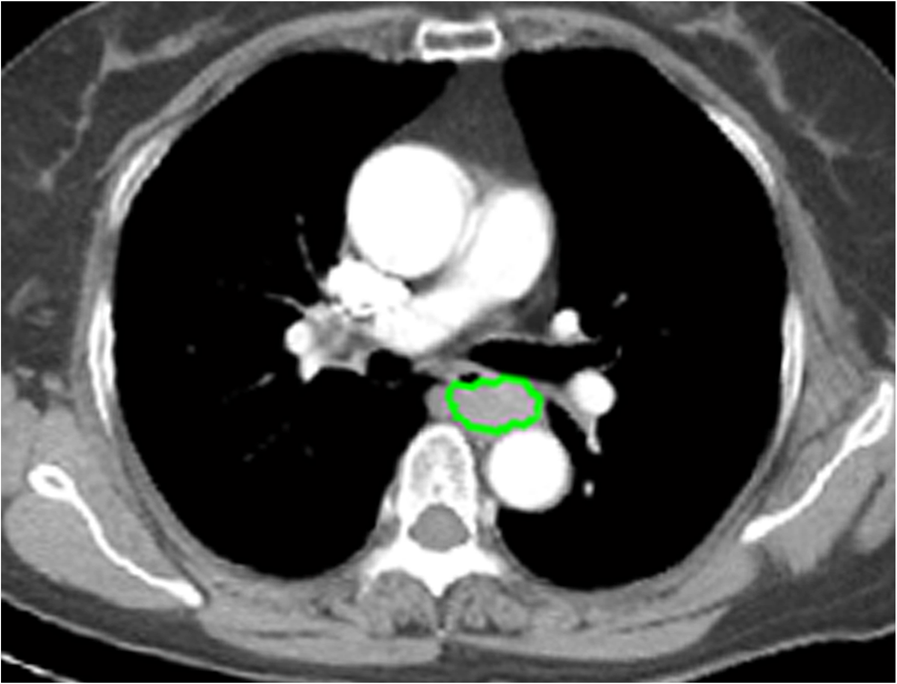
The outlines of oesophageal cancer are manually drawn slice by slice on contrast-enhanced CT data
